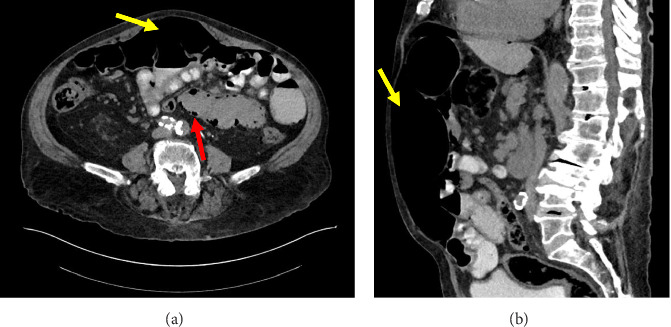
Four-week repeat CT abdomen and pelvis with contrast upon repeat ED presentation: (A) Axial view demonstrating persistent pneumoperitoneum (yellow arrow) and persistent pneumatosis intestinalis (red arrow) of a portion of the small intestine. (B) Sagittal view demonstrating persistent pneumoperitoneum (yellow arrow) spanning from the distal-most aspect of the abdomen to the diaphragm.
